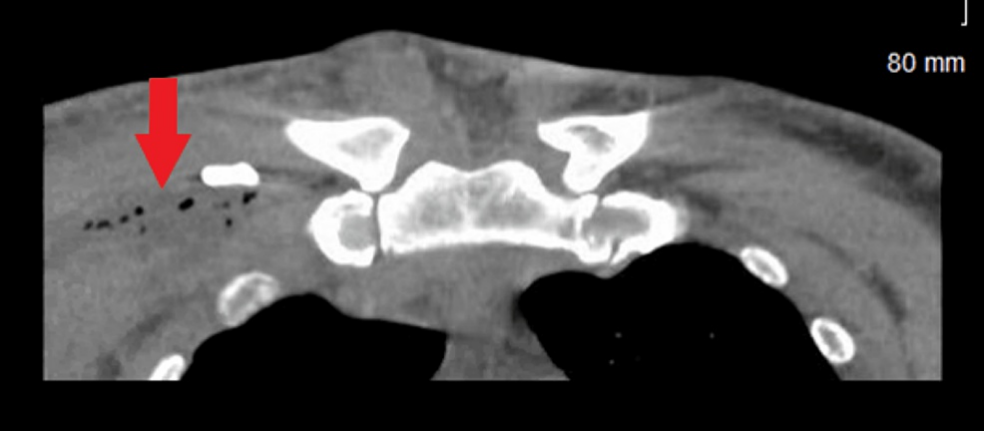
Computed tomography of the chest (coronal view). The red arrow indicates anterior chest wall with developing abscess and mediastinitis.
